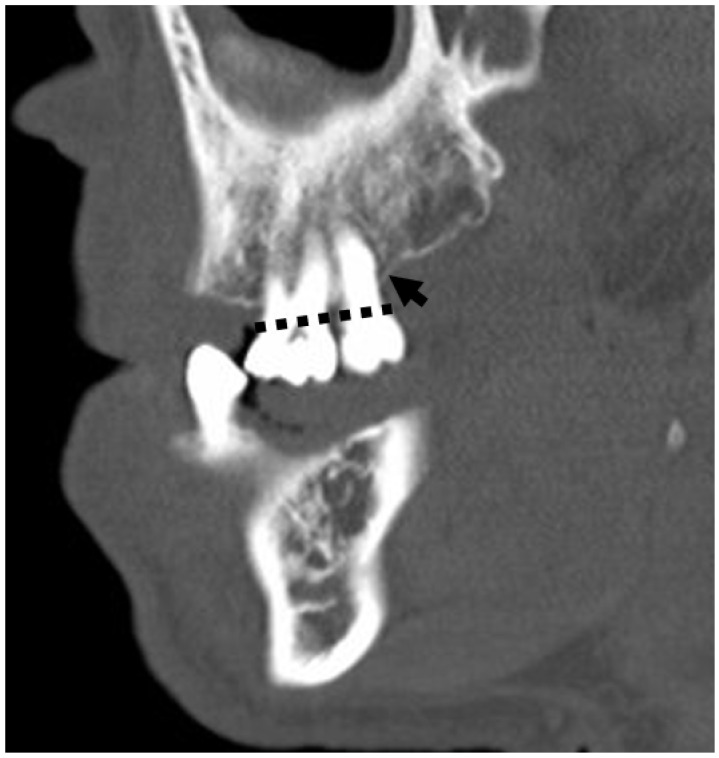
Sagittal section CT demonstrating horizontal bone loss, a sequela of periodontal disease. Note the alveolar crest (arrow) below its normal location at the crown–root junction (dashed line).
